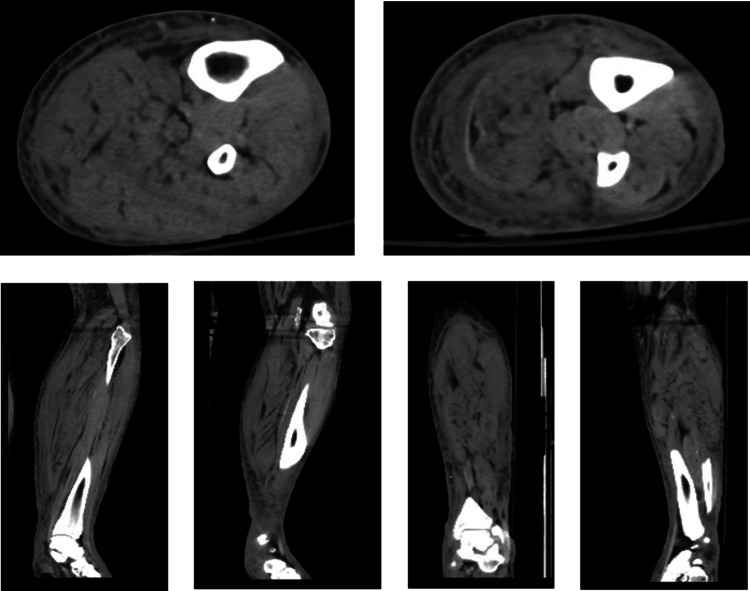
Soft tissue CT images of the coronal, sagittal, and frontal planes of the left lower extremity, confirming edema and subsequent cellulitis
